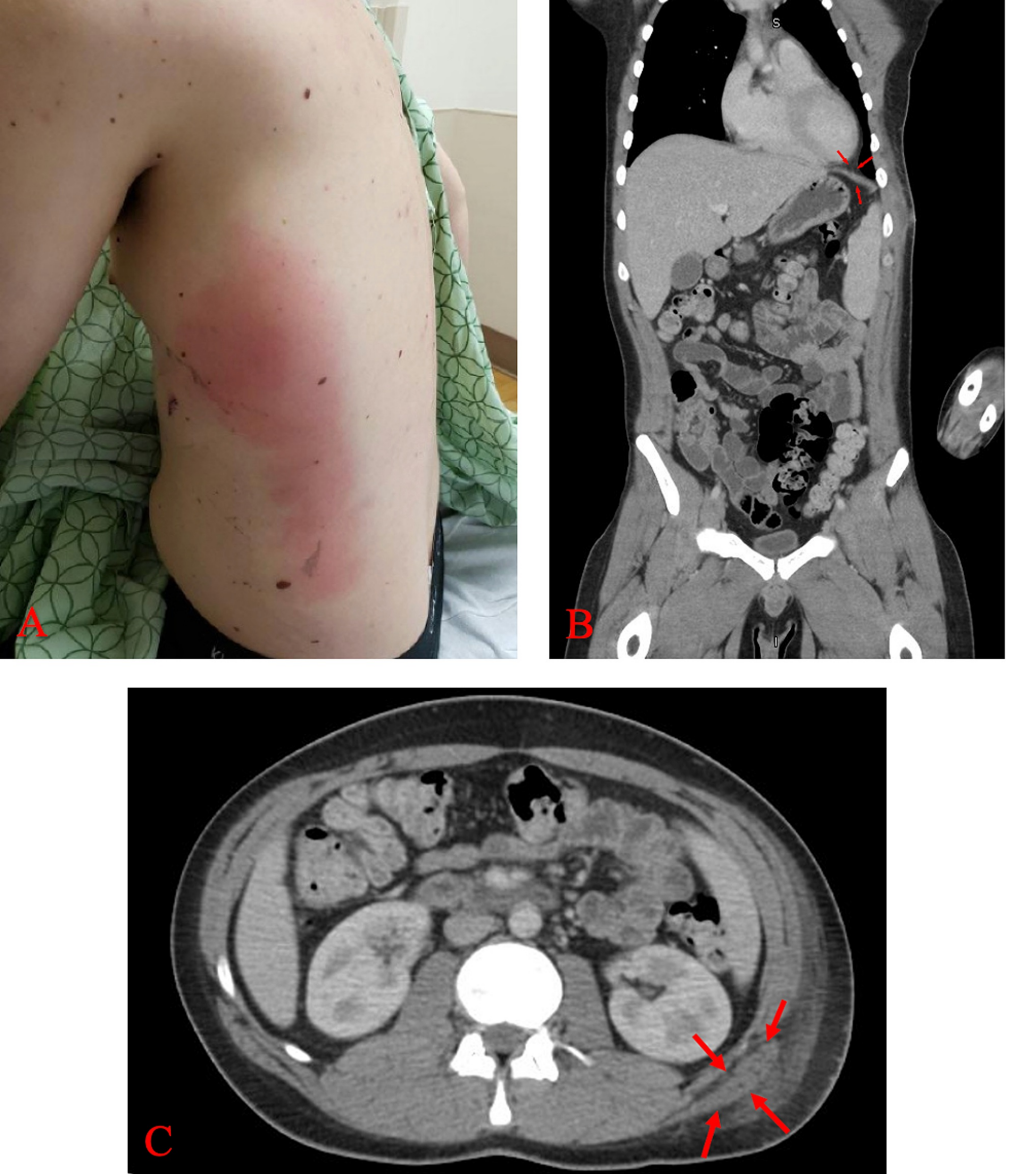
Repeat examination and CT with contrast five days after the initial stab wound. A: There is erythema overlying the area of the initial stab wound, left lateral posterior chest wall, and left flank. B: Fat stranding extending into the subcutaneous fat of the thorax near the left posterior flank is marked with red arrows, indicative of a traumatic diaphragmatic hernia. C: An abscess has formed around the left posterior thorax as indicated by the red arrows.
